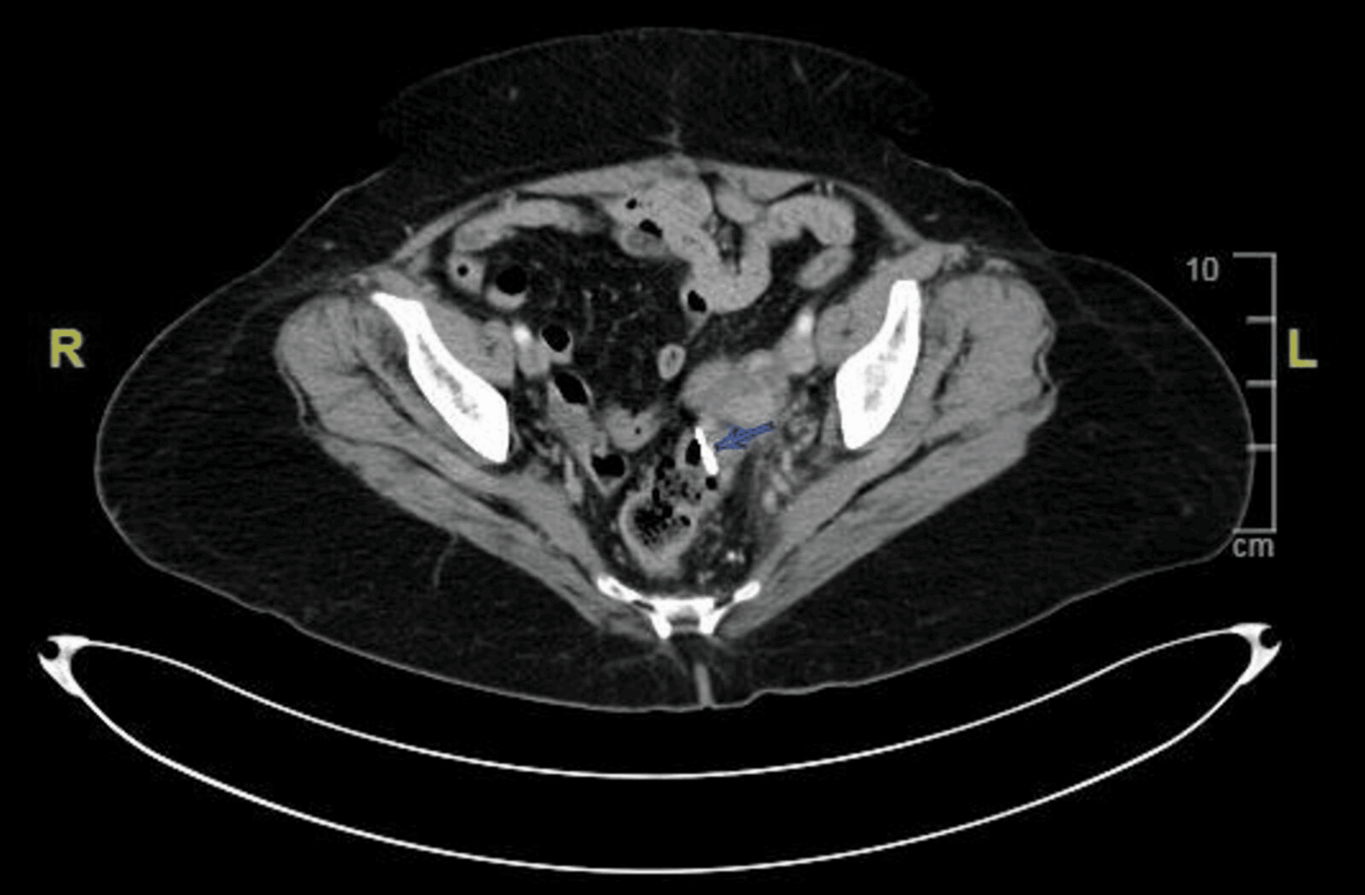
Repeat CT imaging of the abdomen showing hemoclips.
